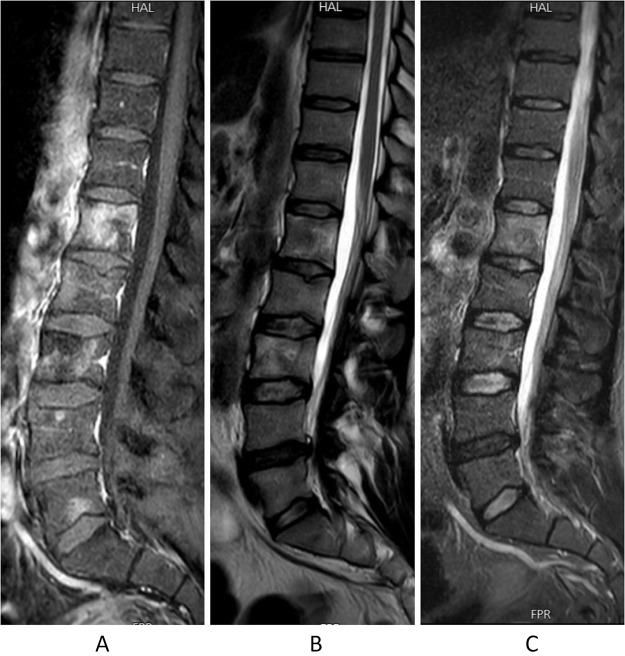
Lumber spine MRI with IV contrast was done and showed the following (blue arrows): (A) sagittal T1 weighted image with IV contrast, (B) sagittal T2-weighted image, and (C) sagittal short tau inversion recovery (STIR). There are multiple heterogeneously enhancing vertebral body lesions with variable signal intensity and high T2WI and short tau inversion recovery (STIR) sequences. There is a cortical disruption in the upper end plate of L2 vertebral body, with no significant decrease in height. L4–L5 level shows dehydrated disc with posterior disc bulge compressing the thecal sac. No significant spinal canal narrowing.
